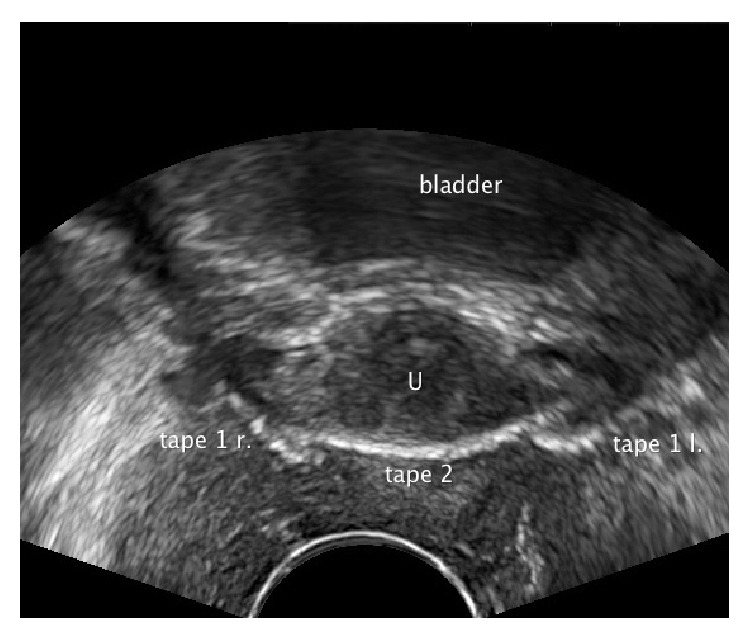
Pelvic floor ultrasound examination in axial plane. The sling was minimally removed vaginally. Both ends however lie still close to the urethra and disturb the second sling in its function: the so-called collision phenomenon. The patient is incontinent. Tape 1 r.: the right end of the first sling. Tape 1 l.: the left end of the first sling. Tape 2: the second sling.
